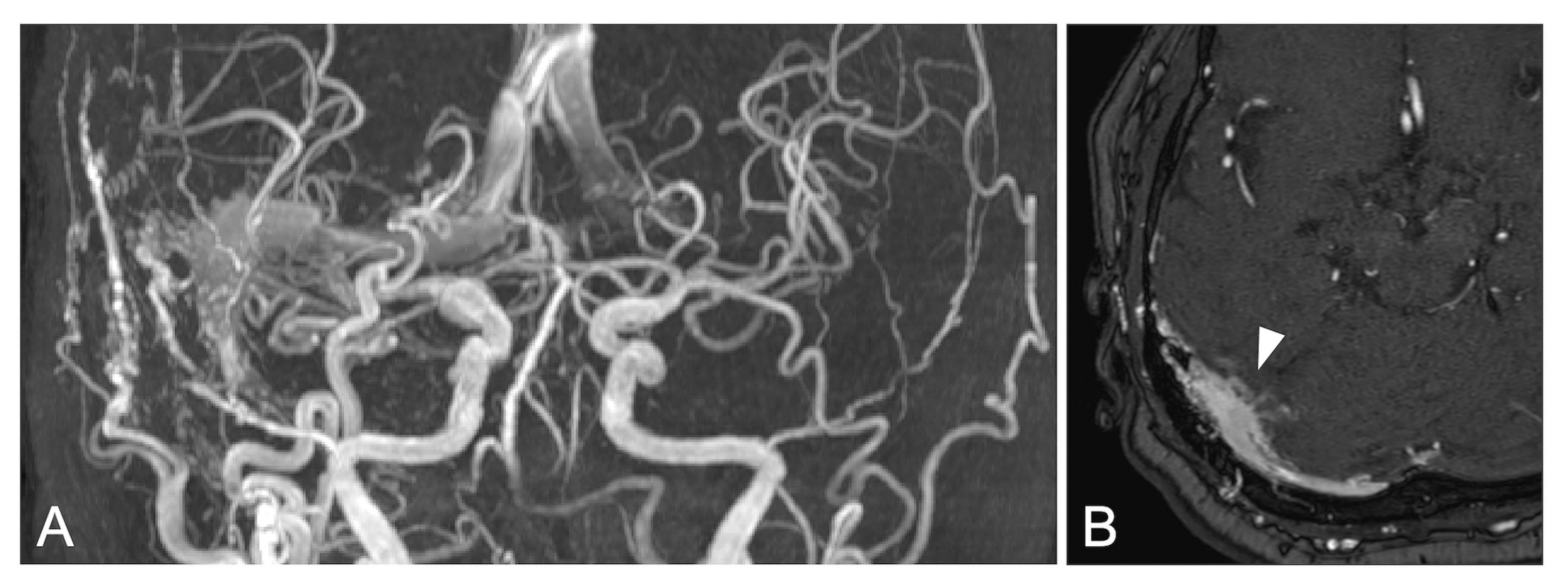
Initial MRI. MRA showing a right transverse-sigmoid sinus dural arteriovenous fistula with both antegrade and retrograde venous drainage (A). The source image of the MR angiography showing cortical venous reflux (arrowhead) (B).
